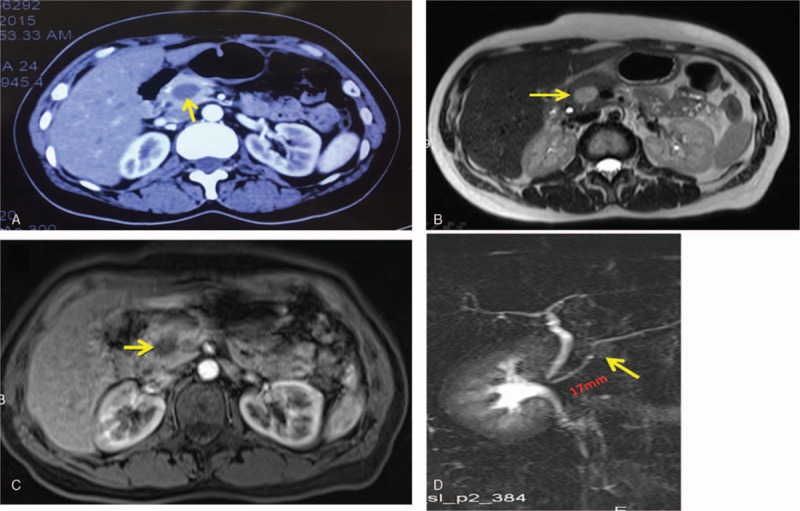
CT, MRI, and MRCP scans. (A) Contrast-enhanced CT and (B, C) contrast-enhanced MRI showed a mixed density tumor in the head of the pancreas (yellow arrow). (D) MRCP showed the main pancreatic duct without dilation, approximately 1.7 cm between the mass (yellow arrow) and Vater's ampulla. CT = computed tomography, MRI = magnetic resonance imaging; MRCP = magnetic resonance cholangiopancreatography.
